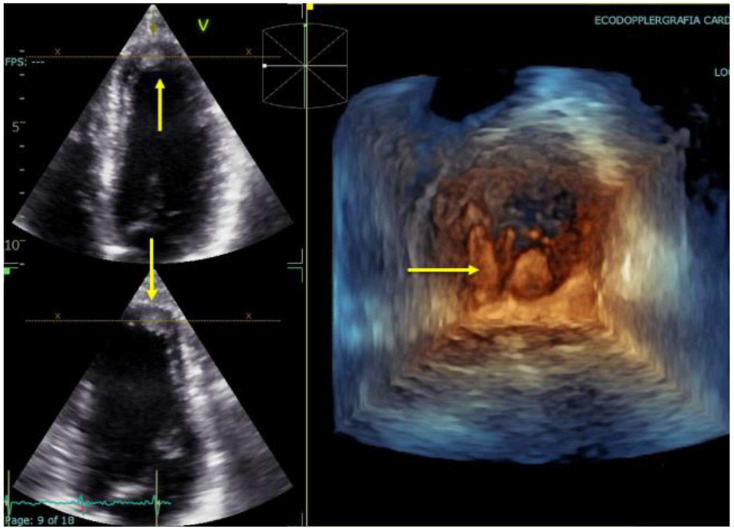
3D ultrasound echocardiography showing akinetic apex with left ventricular sessile thrombus (arrows) into the apex.
